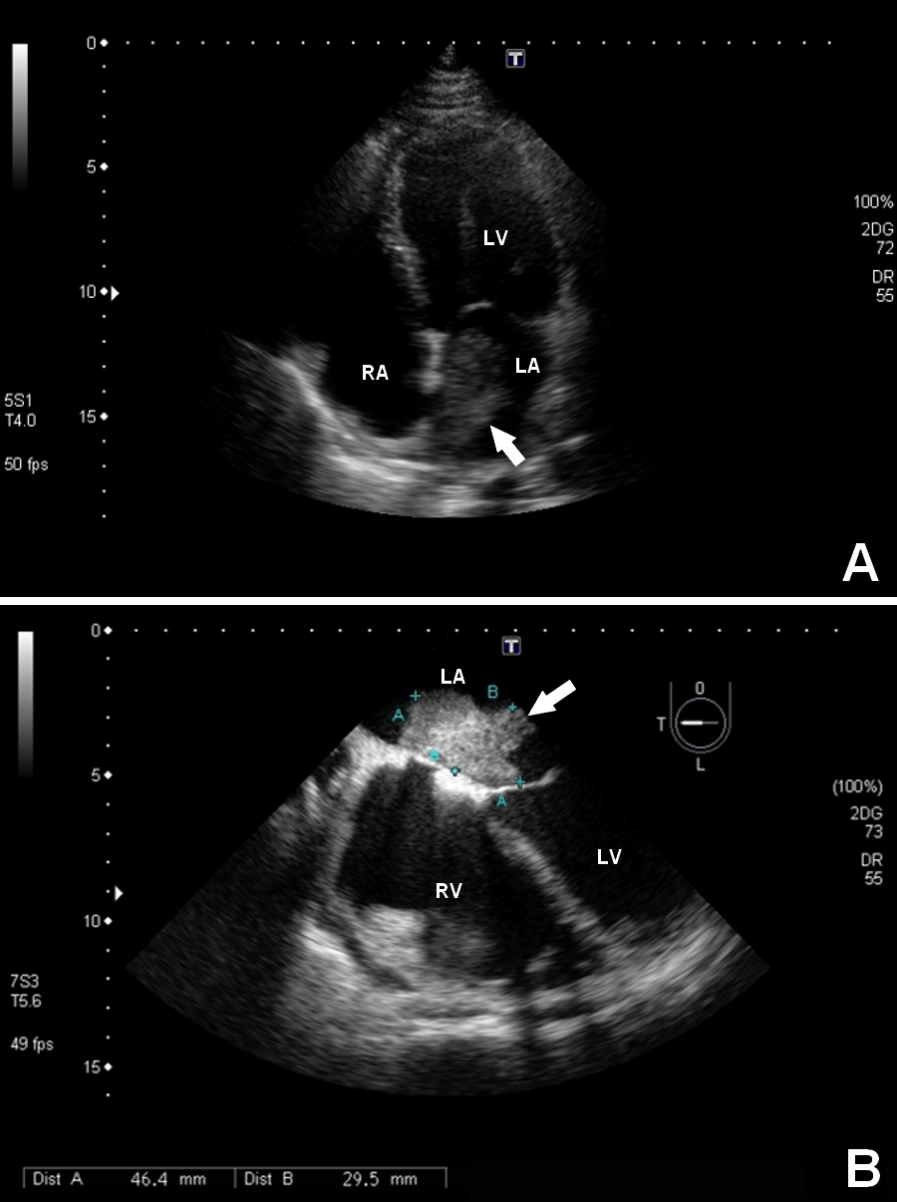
Left atrial myxoma at echocardiography (A) Apical four-chamber view of transthoracic echocardiography showing a large, heterogeneous, irregular, hyperechoic mass inside the left atrium (arrow) (47x30mm). (B) Transesophagic echocardiography showing the myxoma fixed in the interatrial septal wall (arrow) (46.4x29.5mm). LA - left atrium, LV - left ventricle, RA - right atrium, RV - right ventricle.
